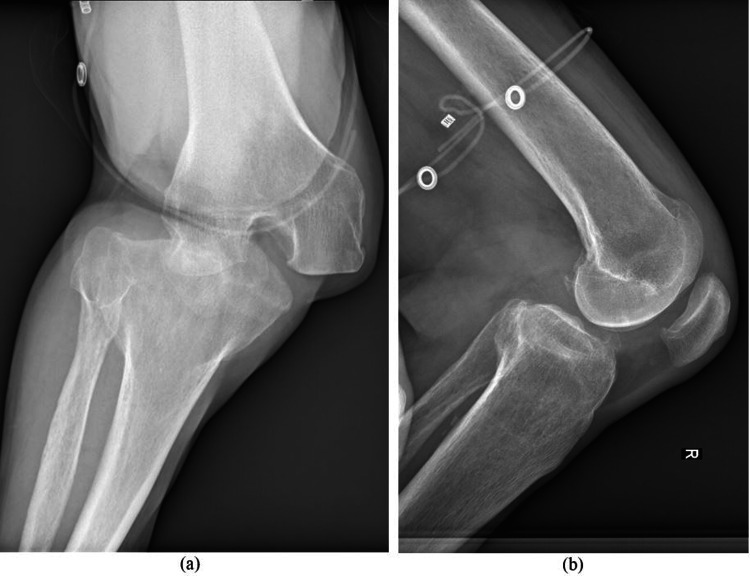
Anteroposterior and lateral radiographs of the right knee.
